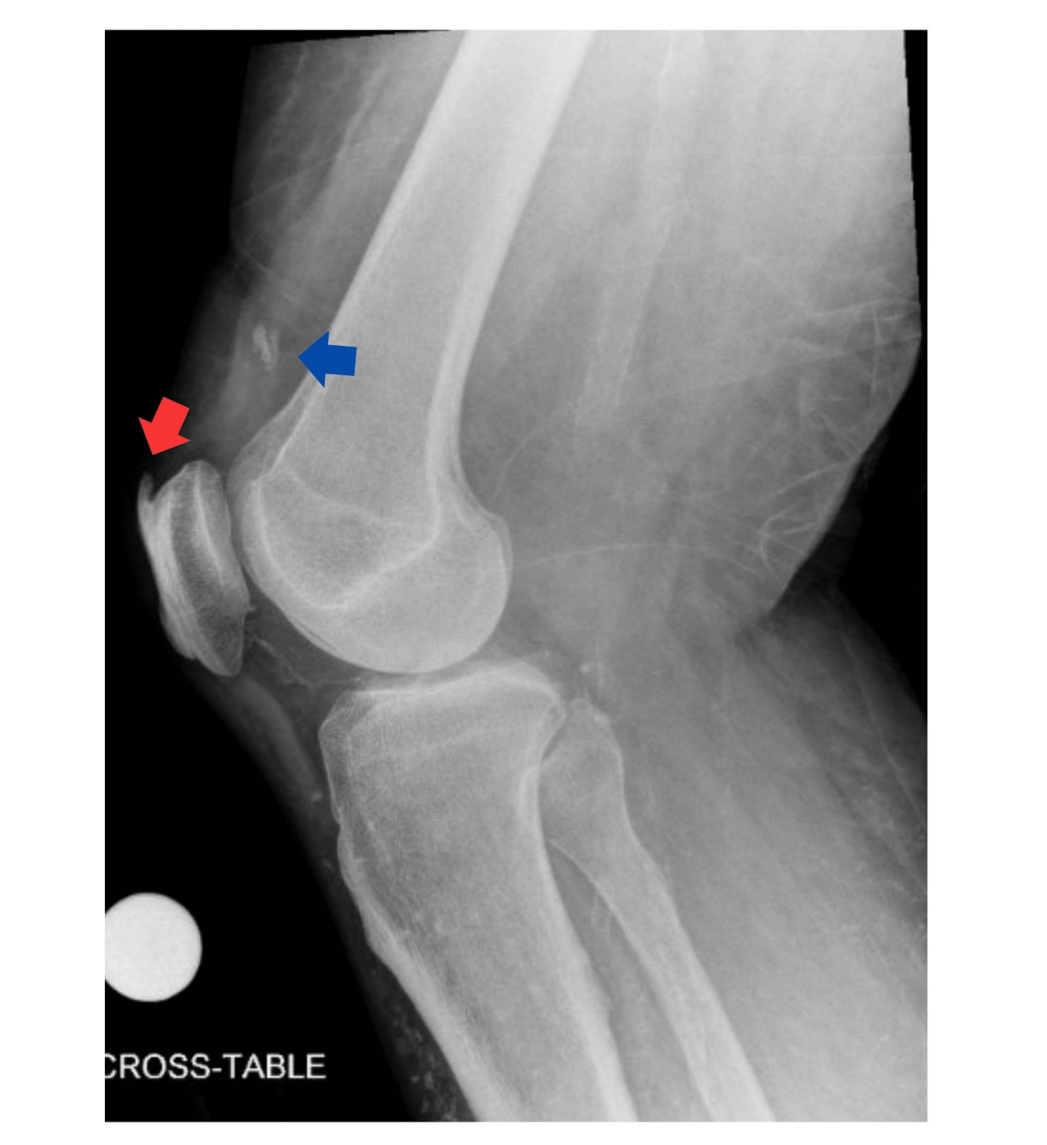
Right Knee X-Ray, Lateral View Lateral view of right knee X-ray taken on initial presentation, demonstrating anterior patellar spur (red arrow) as well as an osseous body overlying the suprapatellar bursa (blue arrow), but no acute fracture.
